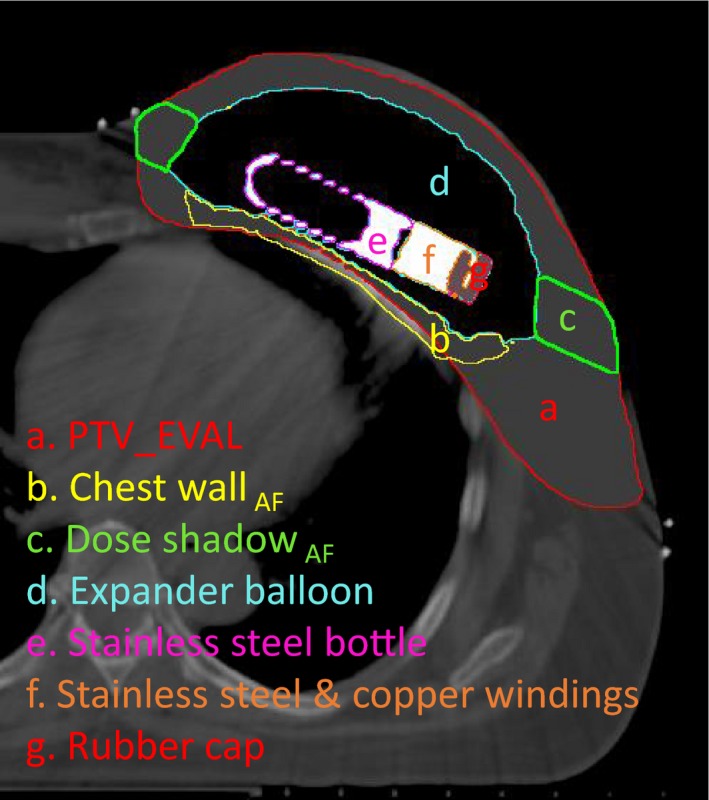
Axial CT of a patient breast with AeroForm TE, with structures created for density overrides and plan evaluation.
